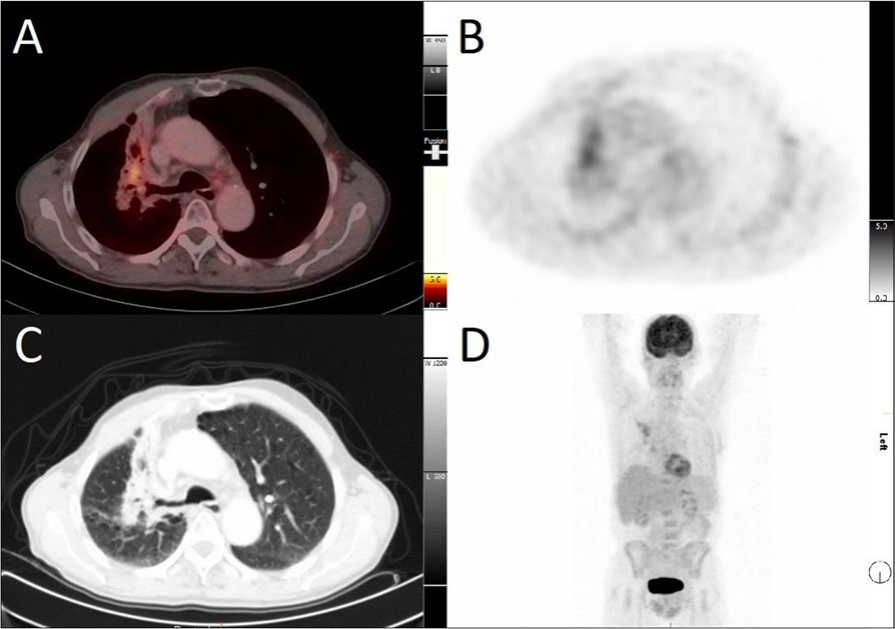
Inflammatory changes in fibrotic tissue after radiation. Axial fused PET/CT image (A), attenuation corrected PET image (B), CT image in lung window (C) and 3D VRT images of the whole body (D) showing fibrotic changes without locoregional tumor recurrence. PET shows diffuse FDG uptake (SUVmax 3.5, SULpeak 2.7) of the right upper lobe 5 years after radiation therapy
